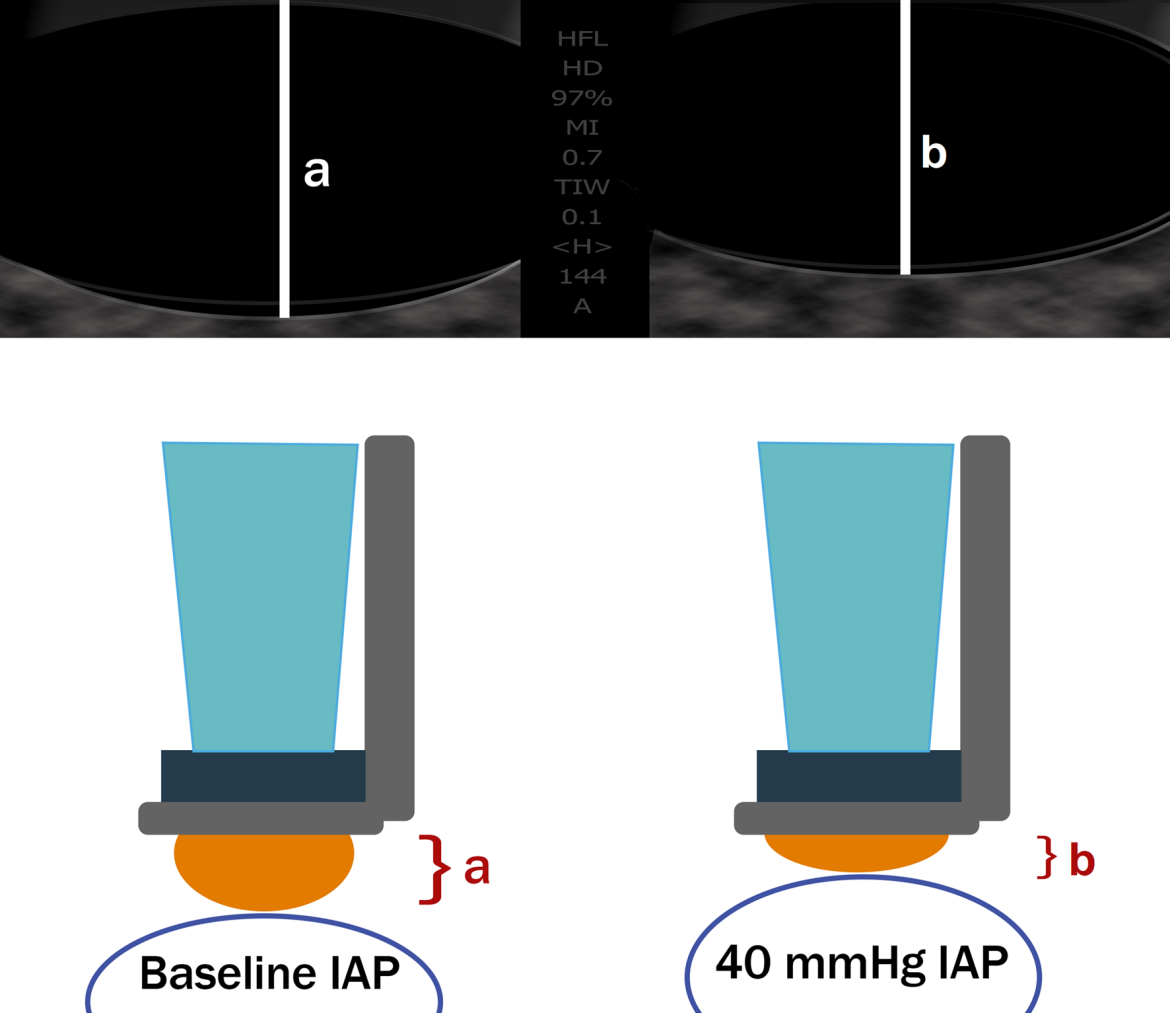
Ultrasound tonometry concept for intra-abdominal pressure assessment. Labels “a” and “b” denote the vertical chamber diameter in two different intra-abdominal pressure (IAP) values (adapted from Bloch et al. [25])
